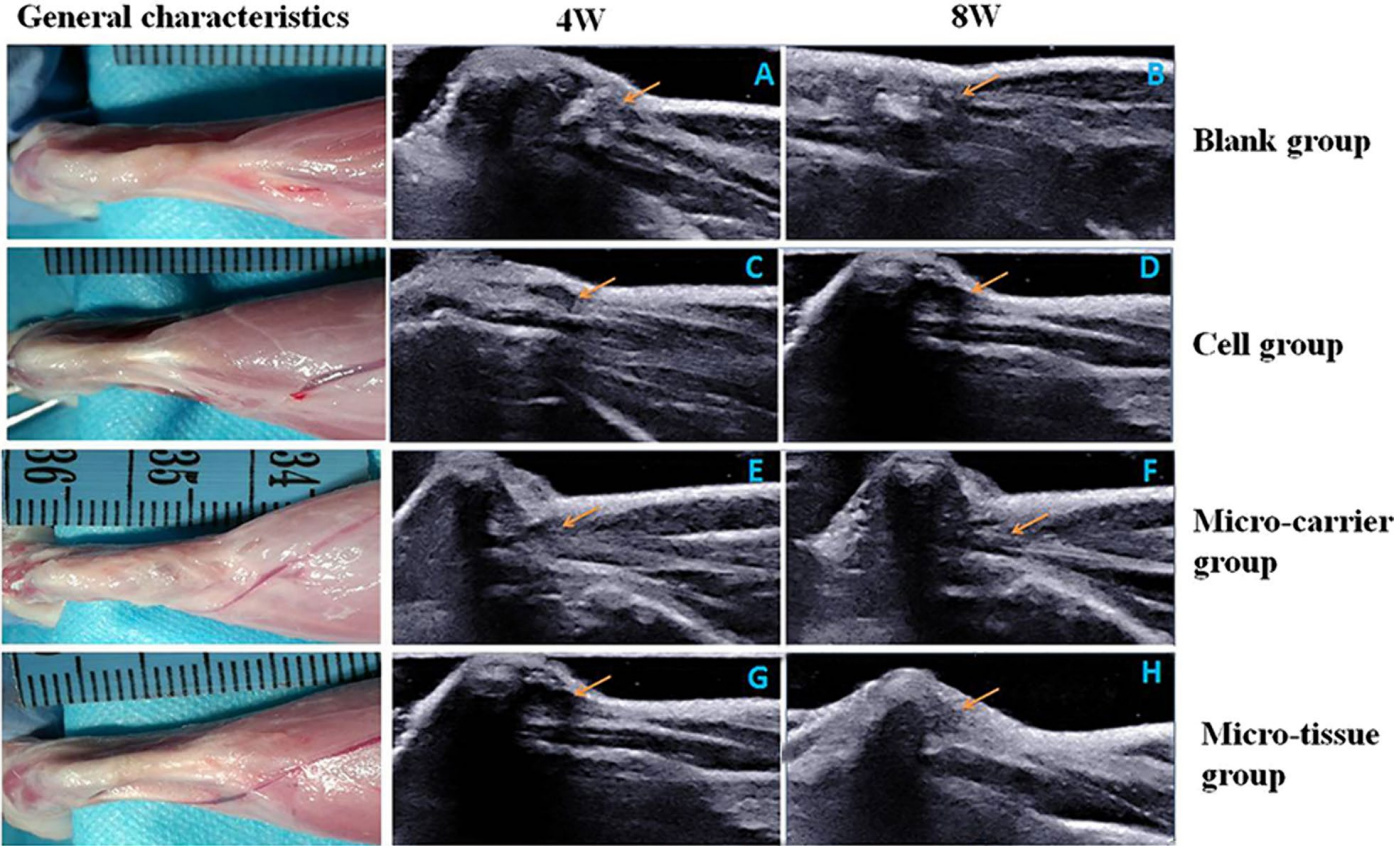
General characteristics at 8 weeks and ultrasound findings. (A-H) Ultrasound findings at 4 and 8 weeks. (Orange arrow indicates Achilles tendon)
